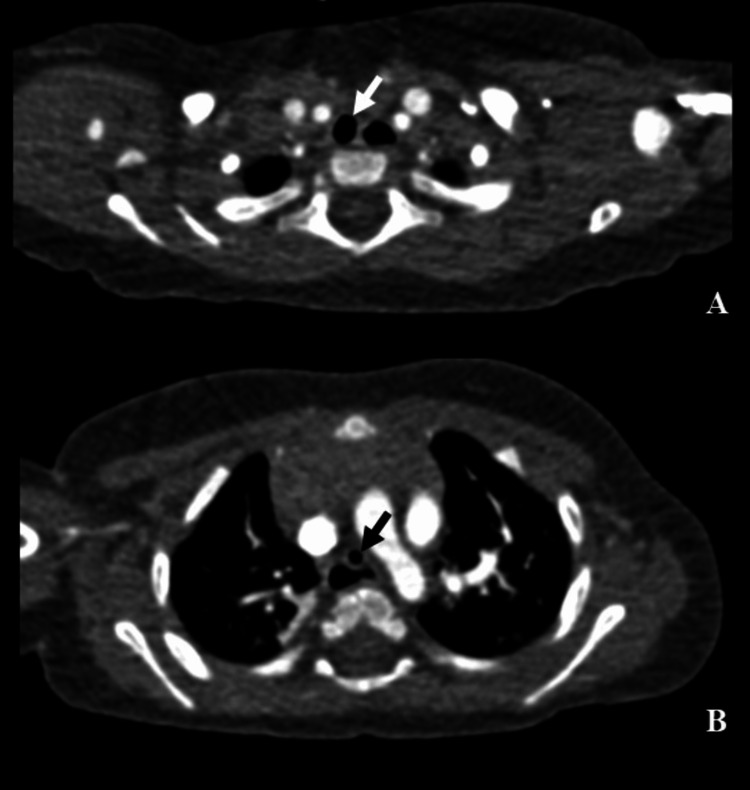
Preoperative computed tomography angiogram of the chest A: Axial view showing a non-stenotic sub-cricoid trachea (arrow). B: Axial view at the thoracic level showing an annular stenotic distal segment of the trachea (arrow).
